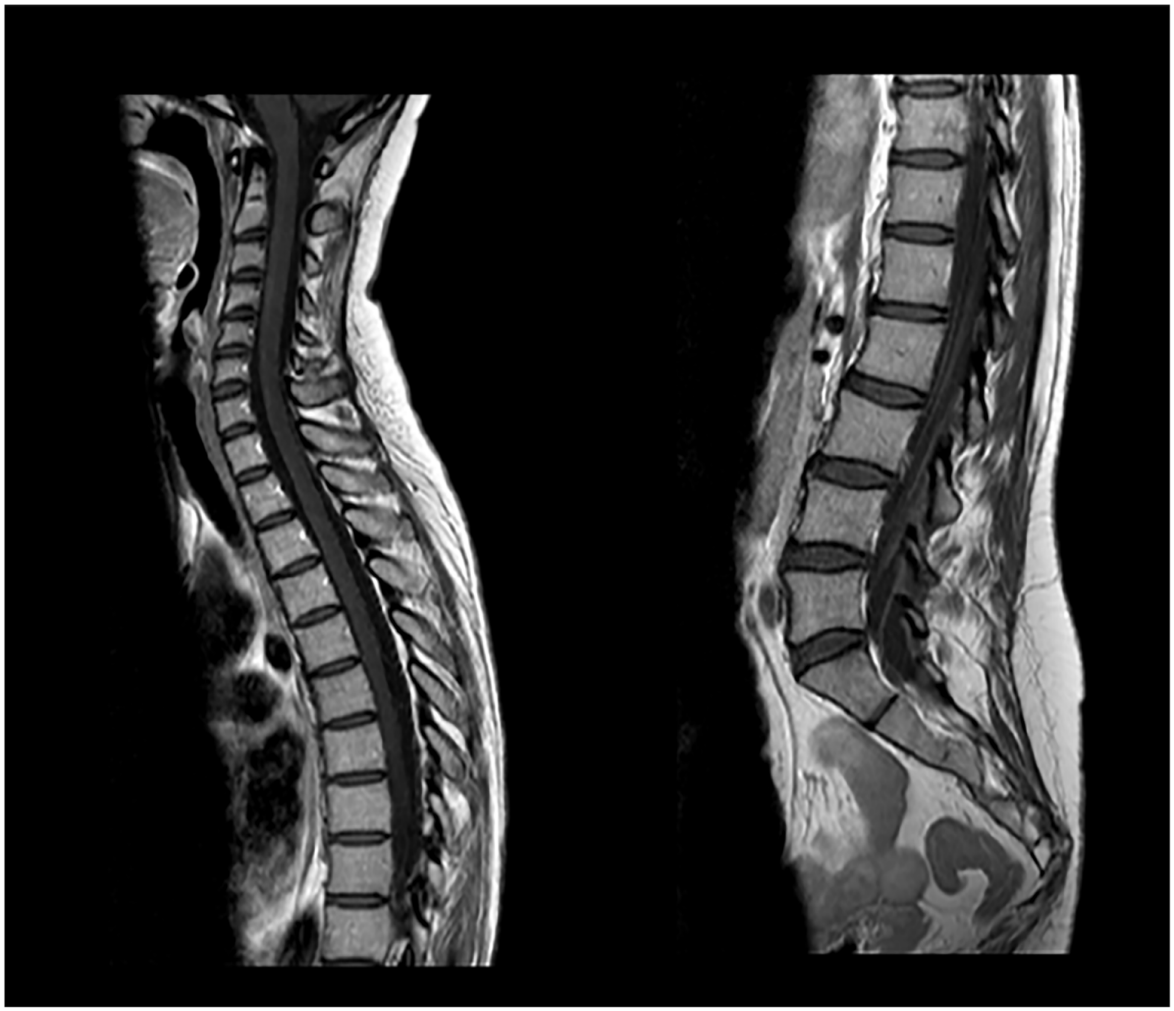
CE and neuraxis MRI response evaluation after 3 cycles of T-DXd. LMC was noticeably reduced (in extension and intensity).
